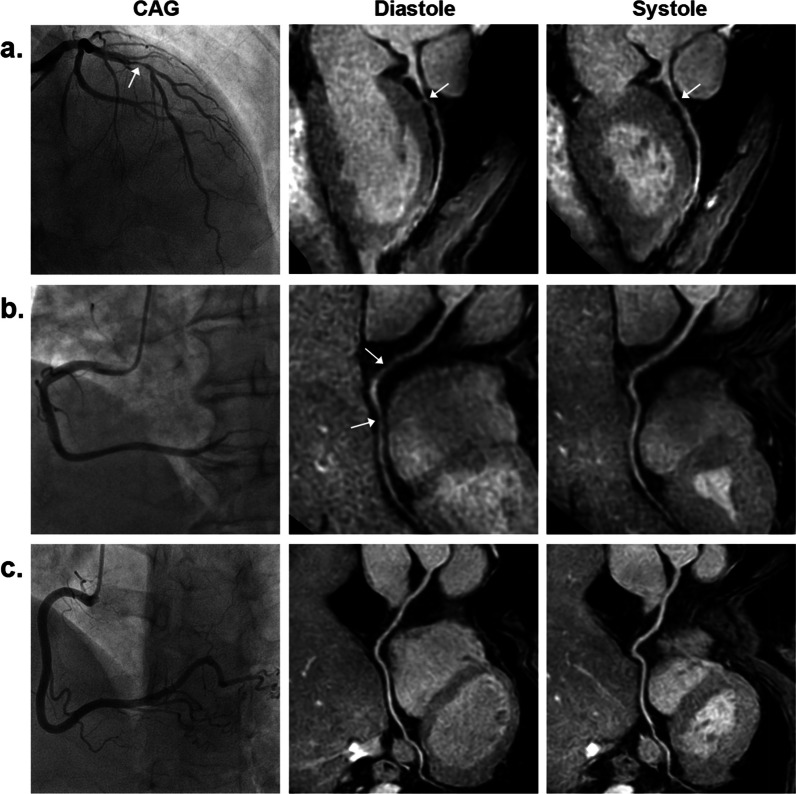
X-ray coronary angiography (CAG) and coronary MR angiography (MRA) at diastole and systole. a Left anterior descending artery (LAD) of a 68-year-old man: CAG shows a significant stenosis (arrow) in the proximal LAD. Coronary MRA at diastole and systole also show a significant stenosis (arrow) in the proximal LAD. For combined coronary MRA analysis, these two location-matched stenosis presented in both modes was determined to be significant. b Right coronary artery (RCA) of a 65-year-old woman: CAG shows a normal RCA without significant stenosis. Coronary MRA at diastole shows false-positive stenoses (arrows) of RCA, which were not observed at coronary MRA at systole, therefore the RCA was defined as having no significant stenosis. c LAD of a 54-year-old woman: CAG shows a normal LAD without significant stenosis. Both coronary MRA at diastole and systole show a normal LAD without significant stenosis, therefore the LAD was defined as having no significant stenosis
